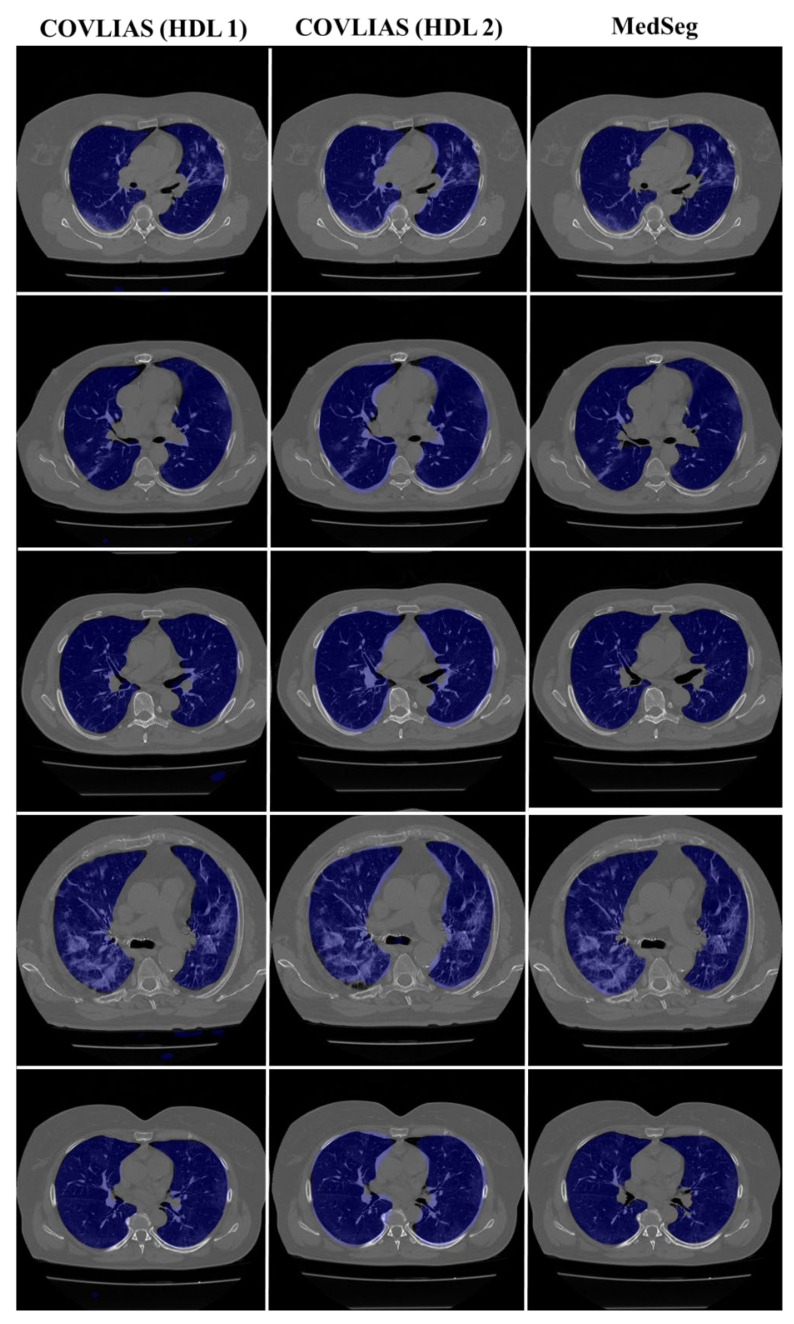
COVLIAS vs. MedSeg: Segmented mask (blue) on the raw CT CROATIA lung image.
